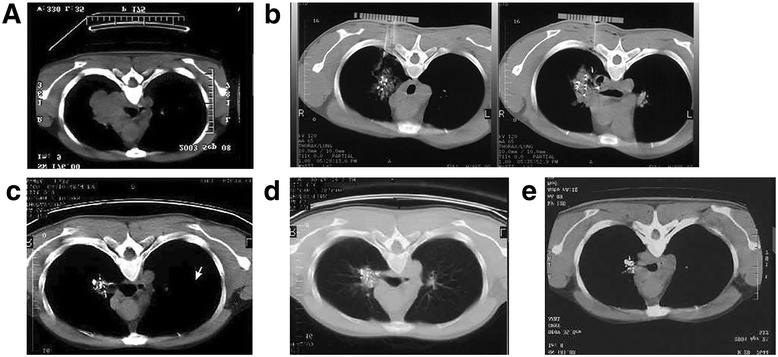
Contrast-enhanced computed tomography (CT) showing the lesion of one typical patient at different time points: pre-operation (a), intra-operation (b), and 1 (c), 3 (d), and 6 (e) months post-operation. The 125I particles gathered slowly after implantation and the lesion fully resolved 6 months after operation
